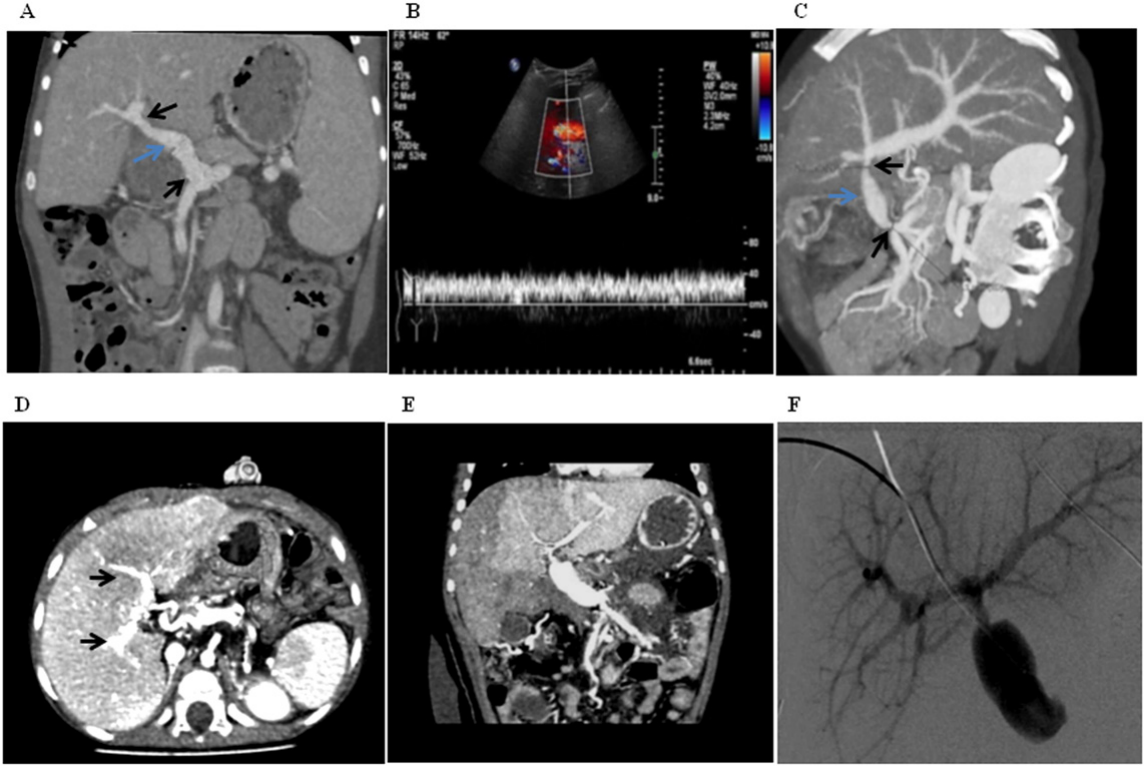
Post-transplant clinical data for the two transplanted patients. (A) Patient 1: Postoperative CT-angiograph, showing the new graft (blue arrow) with good anastomotic sites (black arrows) and well established intrahepatic portal system. (B) The postoperative period was uneventful with good intrahepatic blood flows. The flow velocity showed 30–40 cm/s both in the portal vein and in the hepatic artery. (C) Patient 2: CT-angiography after first transplantation, showing a revascularized portal flow and intrahepatic portal system (blue arrow). At the six-month checkup, the patient had a reduced diameter at both anastomotic sites, (black arrows), however the intrahepatic portal vein system was significantly developed (D). Seven months later the patient received a second graft. (E) CT angiography day after surgery of the second graft revealed poor perfusion of the right portal system. (F) However, after a percutaneous angiography the right portal system was well perfused.
